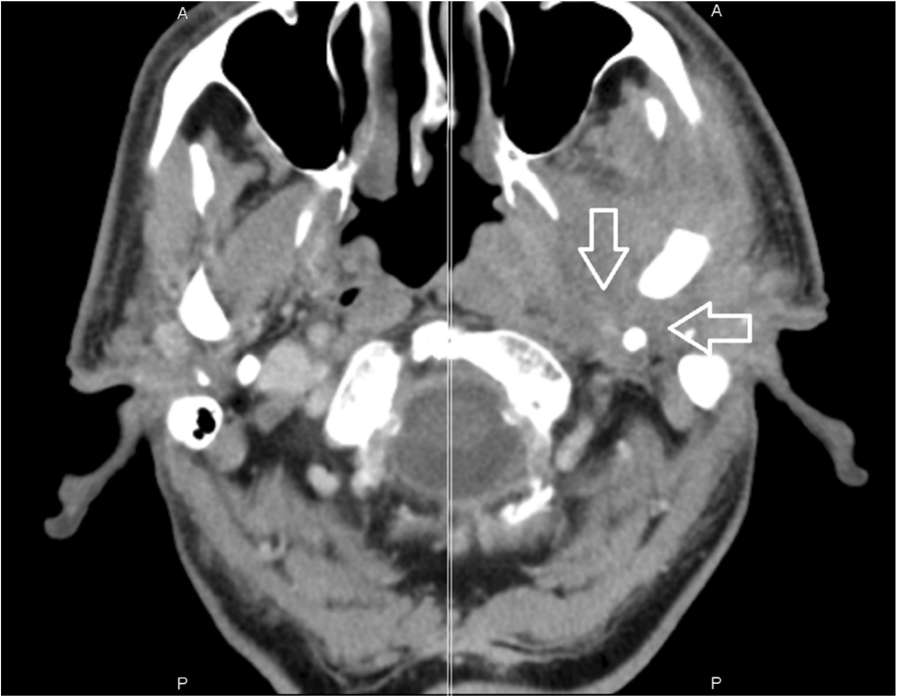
Contrast-enhanced axial CT image of temporal and occipital bones. Oedematous changes in soft tissues adjacent to the stylomastoid foramen on the left side represent the first sign of osteomyelitis spread beyond the temporal bone (arrows)
